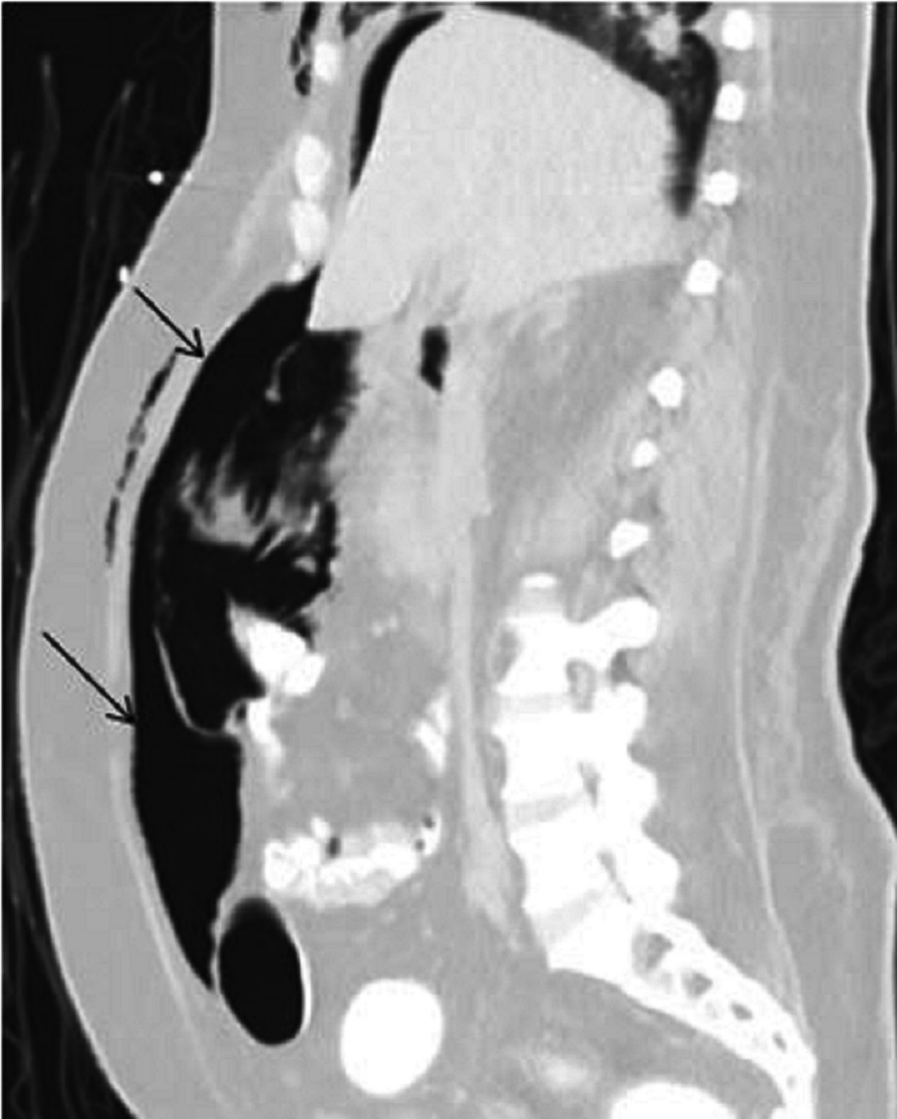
Computed tomography (CT) abdomen with a massive pneumoperitonium
